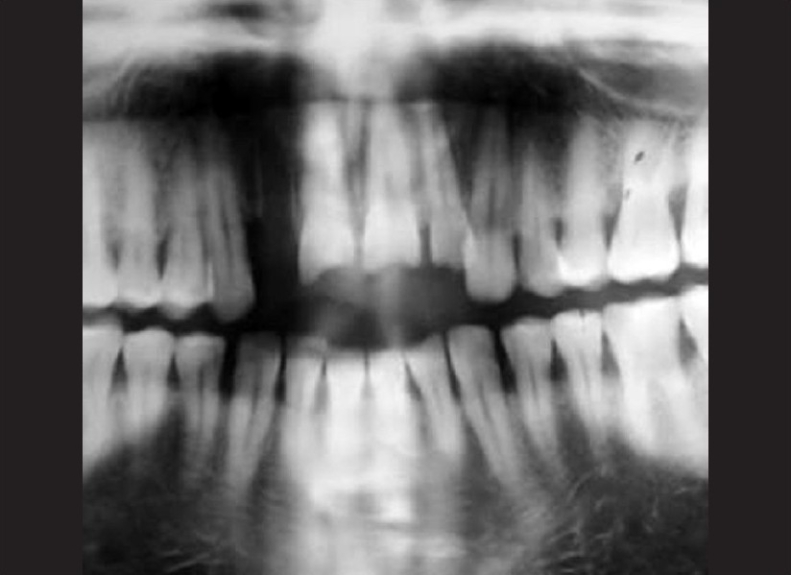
Orthopantomograph of the patient showing the intruded upper incisors with their abnormal relative position with other teeth
